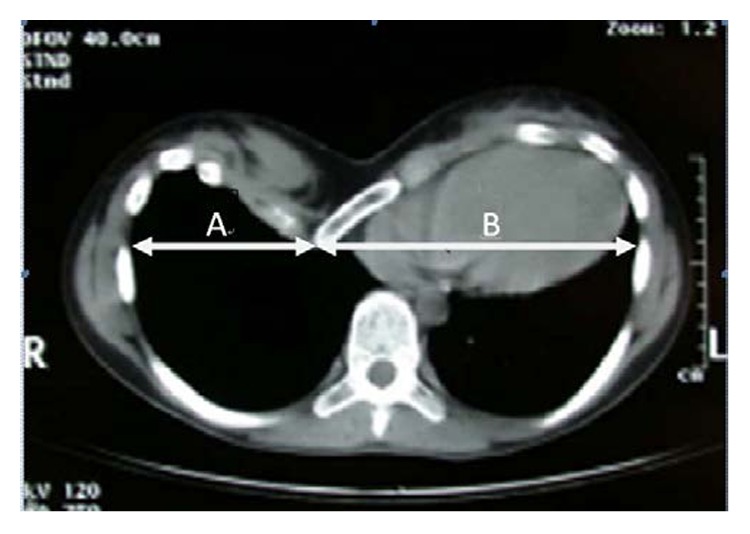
Demonstration of measurements using Mimics on computer. Offset coefficient calculated by A/B×100 is shown in the figure. This CT of a 12-year-old patient exhibited 57 of the offset coefficient; PE apex is on the right chest.
